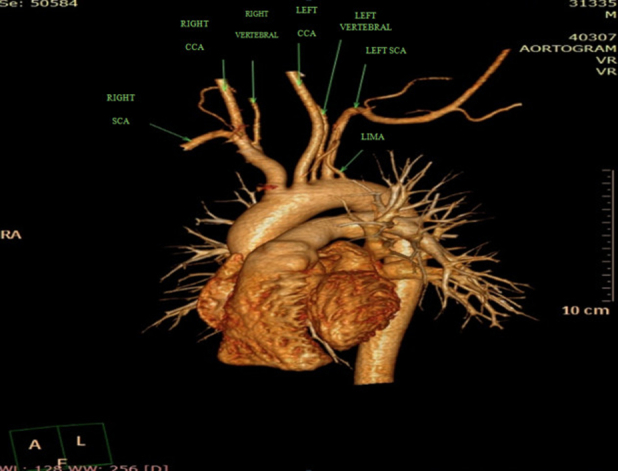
CTA showing the separate origin of left vertebral and LIMA from the aortic arch.
